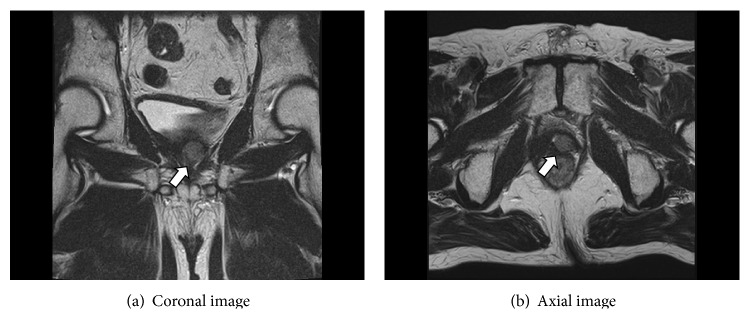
MRI images of local recurrence. T2 weighted image demonstrated a low-intensity area in the left side of prostatic bed.
